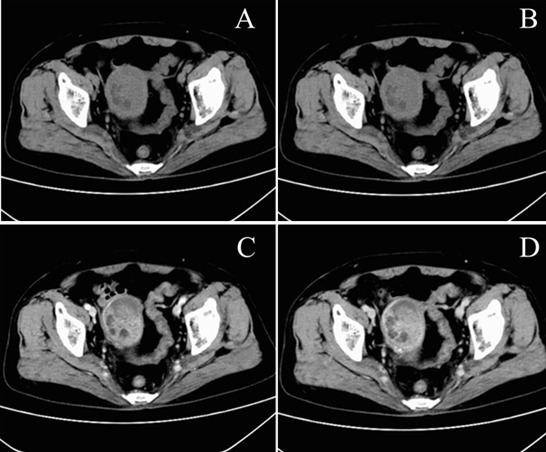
abdominal CT; A) non-contrast: mixed iso-/hypodense uterine mass; B) arterial: minimal enhancement; C) venous: avid solid, absent cystic enhancement; D) delayed: persistent solid, no cystic enhancement
